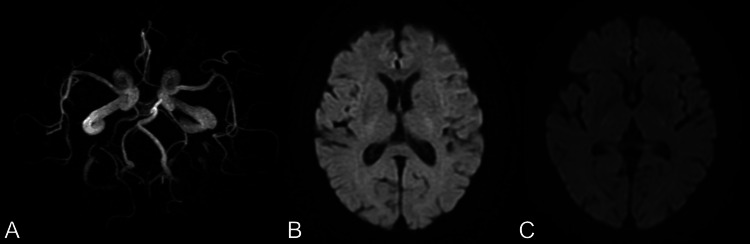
The brain magnetic resonance imaging (MRI) of the present case A) Preoperative magnetic resonance angiography (MRA); B) preoperative diffusion-weighted image (DWI); C) postoperative diffusion-weighted image (DWI) Preoperative MRA showed no defects in the major vessels and the circle of Willis. Preoperative DWI showed no significant lesions except for old lacunar infarction. Postoperative DWI showed no obvious new lesions.
